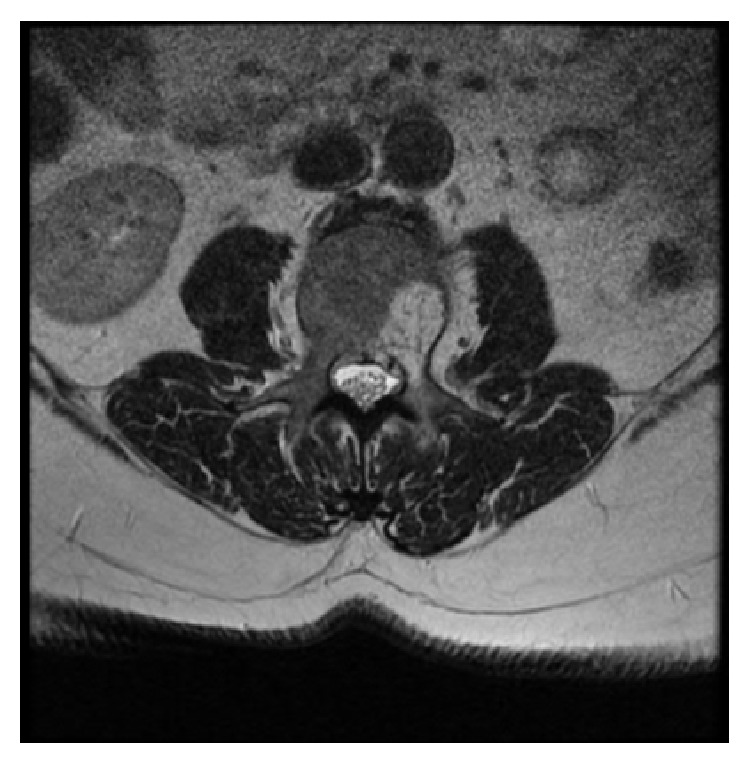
MRI of the spine; T2 weighted images demonstrating hyper intense skeletal lesions.
